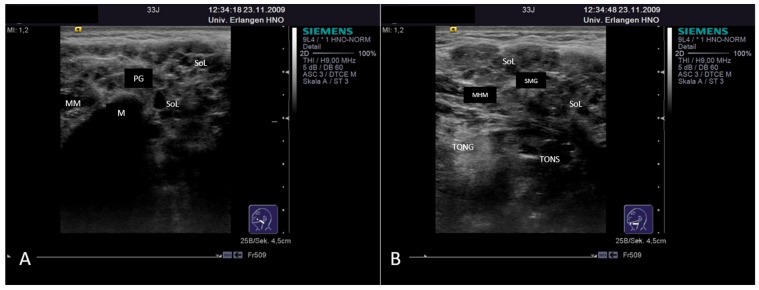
(A,B): Ultrasound findings at the advanced stage of M. Sjögren in the left GP (A) and left SMG (B). The parenchyma is markedly heterogeneous and replaced by hypoechoic to anechoic spot-like formations as less parenchyma is recognizable. Hypoechoic areas appear more solid and may correspond to an infiltration of lymphatic cells, anechoic to sialectatic ducts after atrophy of the acinic cells. The glands are enlarged. Abbreviations: PG, parotid gland; SMG, submandibular gland; M, mandible; MM, masseter muscle; MHM, mylohyoid muscle; TONG, tongue; TONS, tonsil; SoL, space-occupying lesion.
